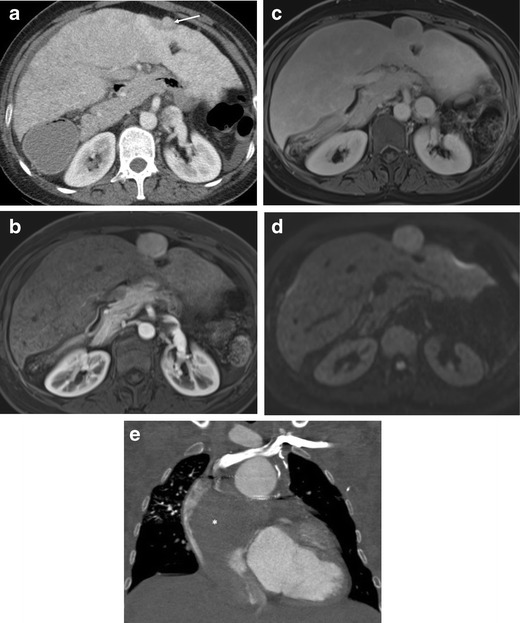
Hepatocellular carcinoma with extensive tumour thrombus in a post-Fontan patient. A 38-year-old woman with previous right atrial isomerism and pulmonary atresia. a Axial CT image in the portal venous phase demonstrates a 1.1-cm iso-attenuated nodule causing surface contour abnormality in segment 2 of the liver (*arrow*). This lesion was initially reported as regenerative nodule. b-d On 6-month follow-up MRI, the lesion increased to 3.4 cm, showing arterial enhancement (b), washout in 3-min delay (c), and strong diffusion restriction in single-shot echo-planar diffusion-weighted image (*b* = 1,000 s/mm^2^) (d). Biopsy confirmed the lesion as HCC and the lesion was successfully treated with radiofrequency ablation (RFA). e Local recurrence of segment II in the dome HCC directly invaded the inferior vena cava (not shown) and subsequently an extensive tumour thrombus developed and filled the inferior vena cava, the right atrium (*asterisks*) and extended to the left main pulmonary artery
